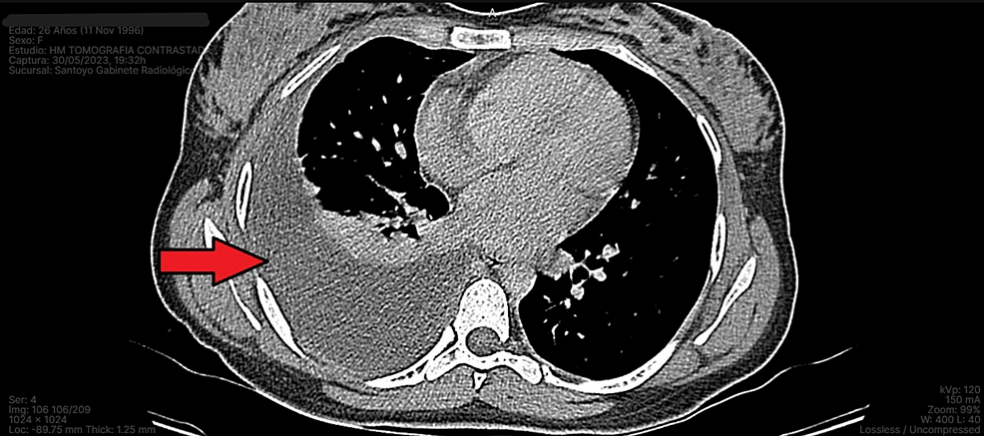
CT scan of the chest mediastinal window showing right-sided pleural effusion
